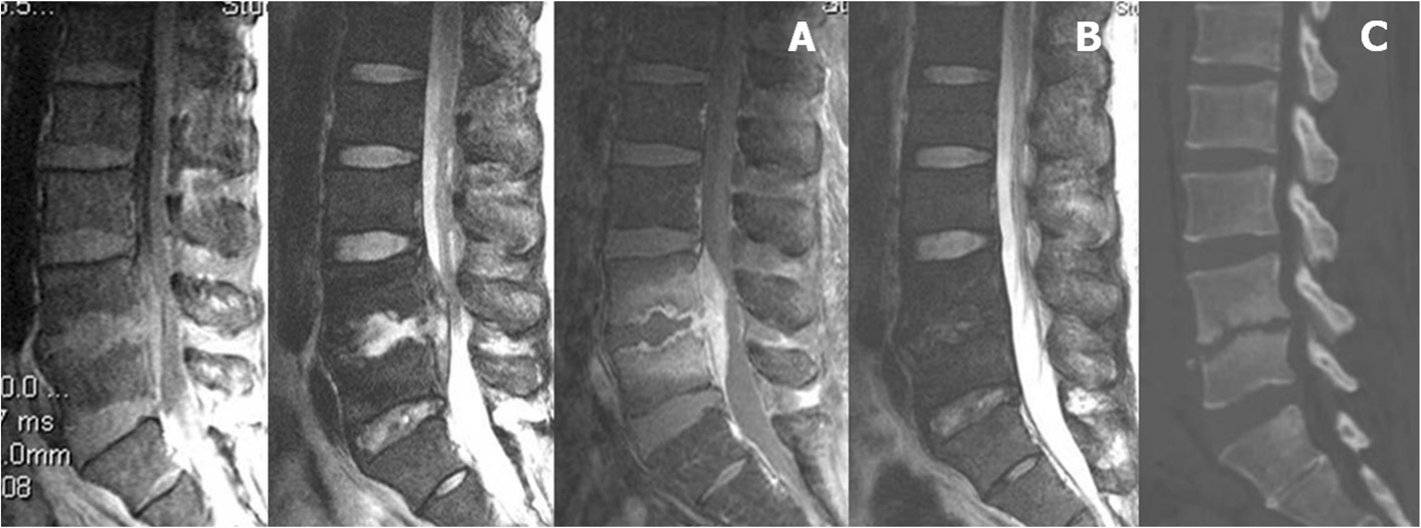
Epidural abscess treated by PEDI. L4-5 infectious spondylitis was diagnosed in a male patient. Sagittal T1- and T2-weighted and contrast magnetic resonance imaging (MRI) showed L4-5 epidural abscess with compression of neural elements (A). After PEDI treatment, sagittal T2-weighted MRI at 6 months follow-up demonstrated the disappearance of the abscess (B). Lateral computed tomograph revealed L4-5 disc space narrowing leading to spontaneous fusion (C).
